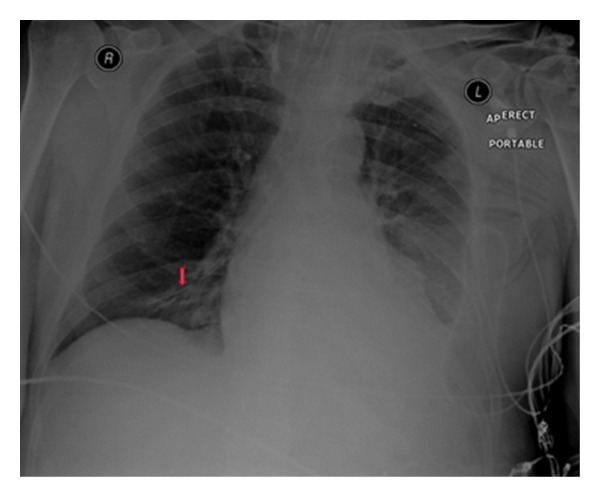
The quality of a portable AP radiograph is not always optimal because of the technical factors prevailing. The tip of the NGT in the lower lobe bronchus is obscured by lung markings (arrow).
